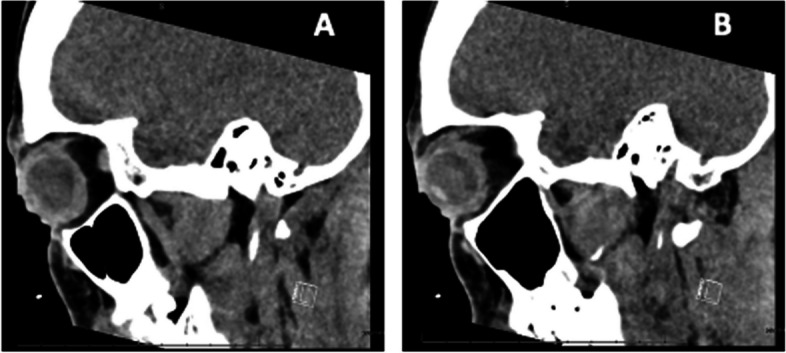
A: CT of the right eye shows circumferential thickening of the walls of the globe B: CT of the left eye also demonstrates circumferential thickening the walls of the globe
